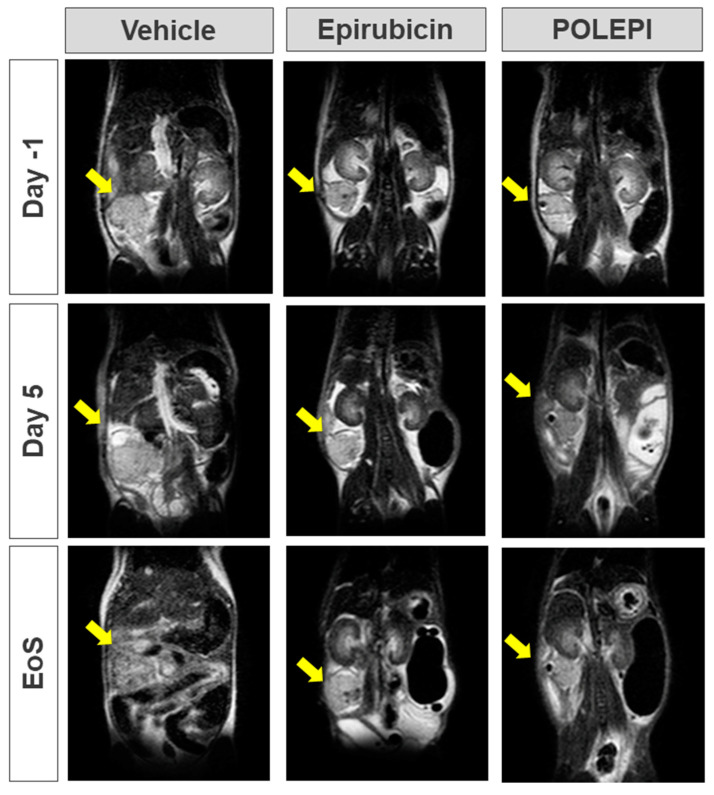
Orthotopic PDX tumor in Certis Orthotopic ovarian model visualized via MRI. Yellow arrow indicates tumor. EoS: Day 7–9; vehicle: AN#001 for Day-1, Day 5 and EoS; epirubicin: AN#022 for Day-1, Day 5 and EoS; and POLEPI: AN#011 for Day-1, Day 5 and EoS.
